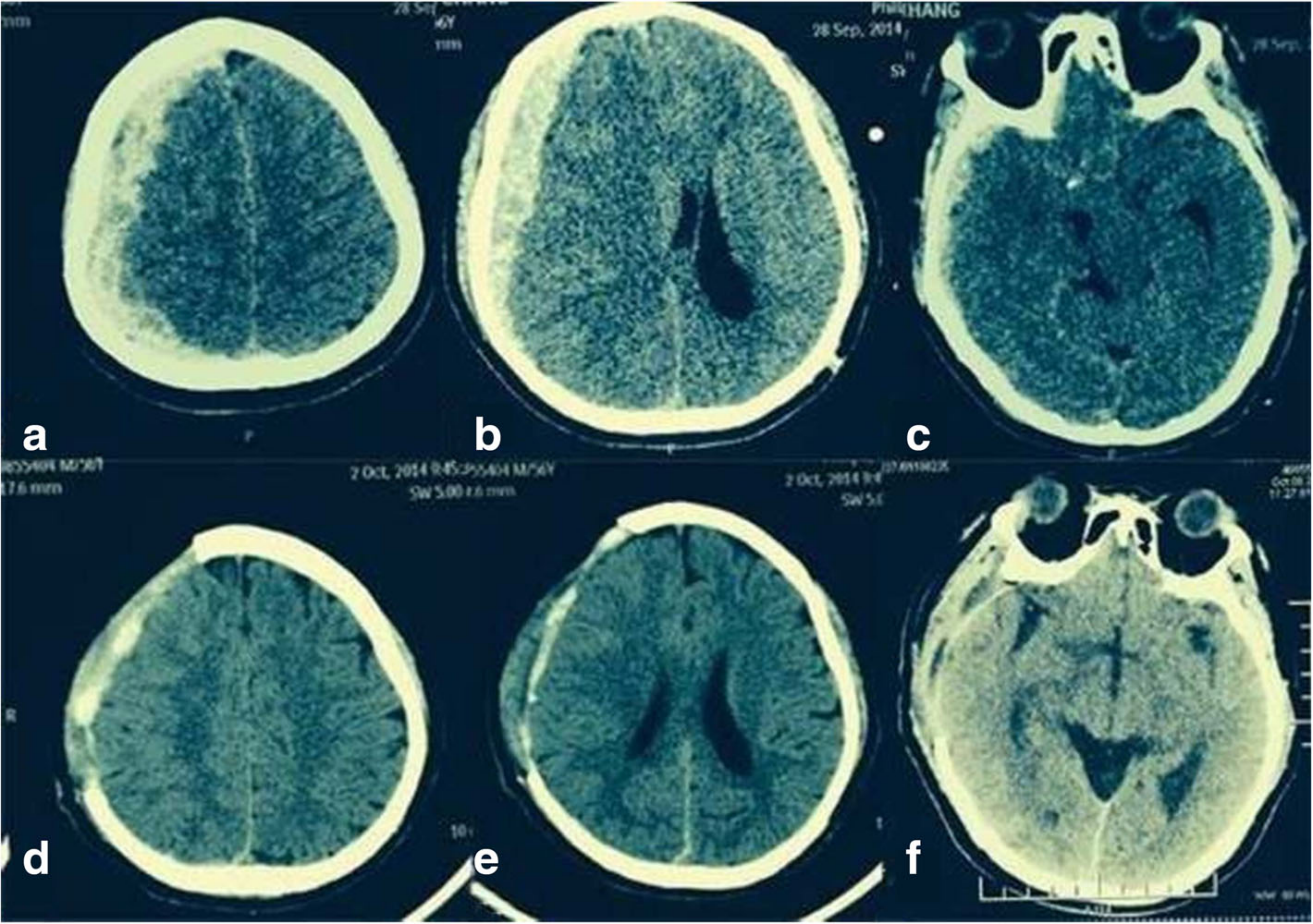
a, b, c CT scans before the operation. SDH was detected at the parietal and frontotemporal level of the right side. d, e, f CT scans after the operation. SDH had disappeared
